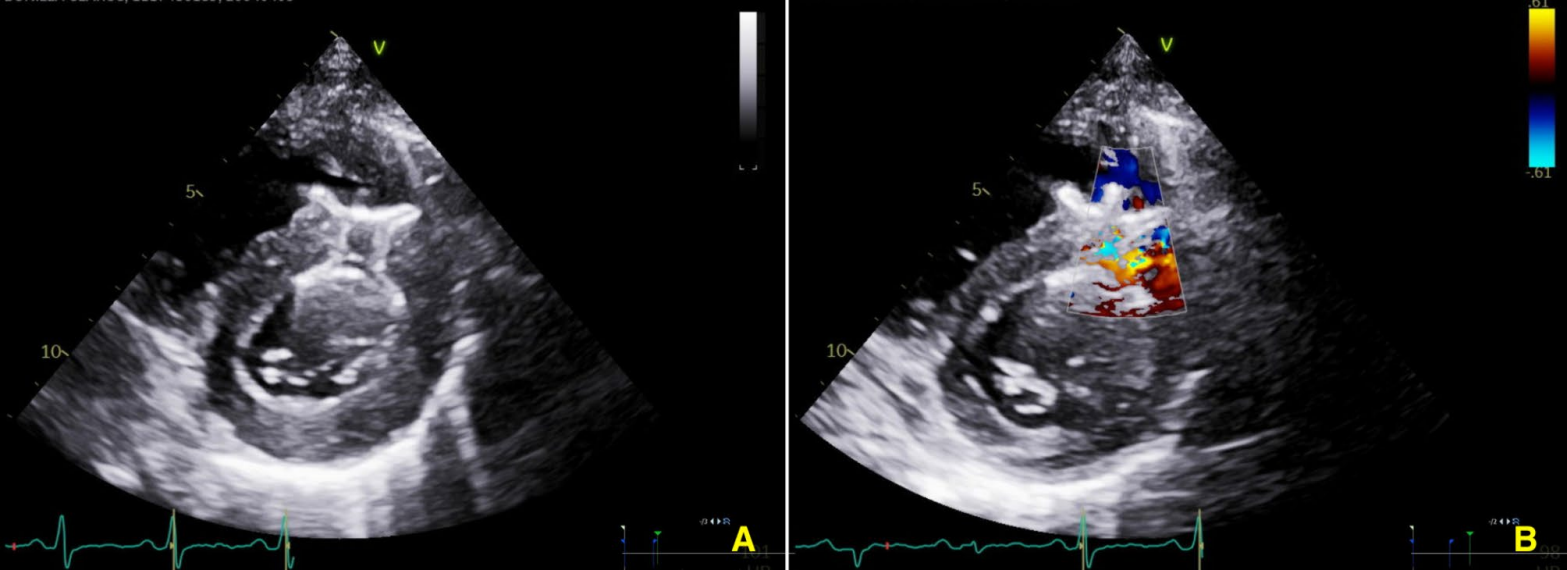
Transthoracic echocardiogram. Parasternal short-axis view A. A well-positioned VSD closure device is observed in the middle segment of the anterior septum. B. Color Doppler: Unidirectional left-to-right residual shunt, with a large decrease in flow compared to the preprocedural study
